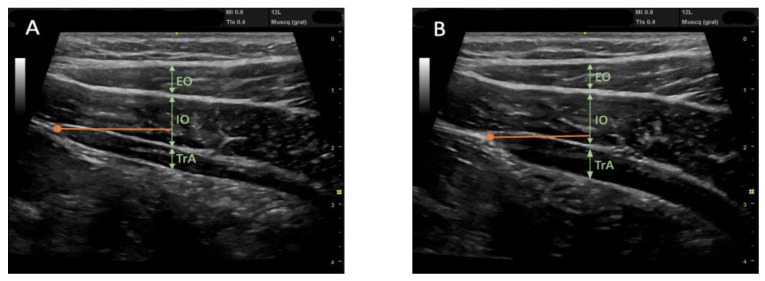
Ultrasound images of the lateral abdominal wall musculature showing the 3 muscle layers from top to bottom: External Oblique (EO), Internal Oblique (IO), and the deepest, Transverse Abdomen (TrA); separated from each other by intermuscular connective tissue (hyperechoic lines). (A) Image at rest. (B) An image taken during muscle activity showing a change in muscle thickness. Measurements are always taken at the same point, 2 cm (the orange mark) from the insertion of the TrA in the RA.
